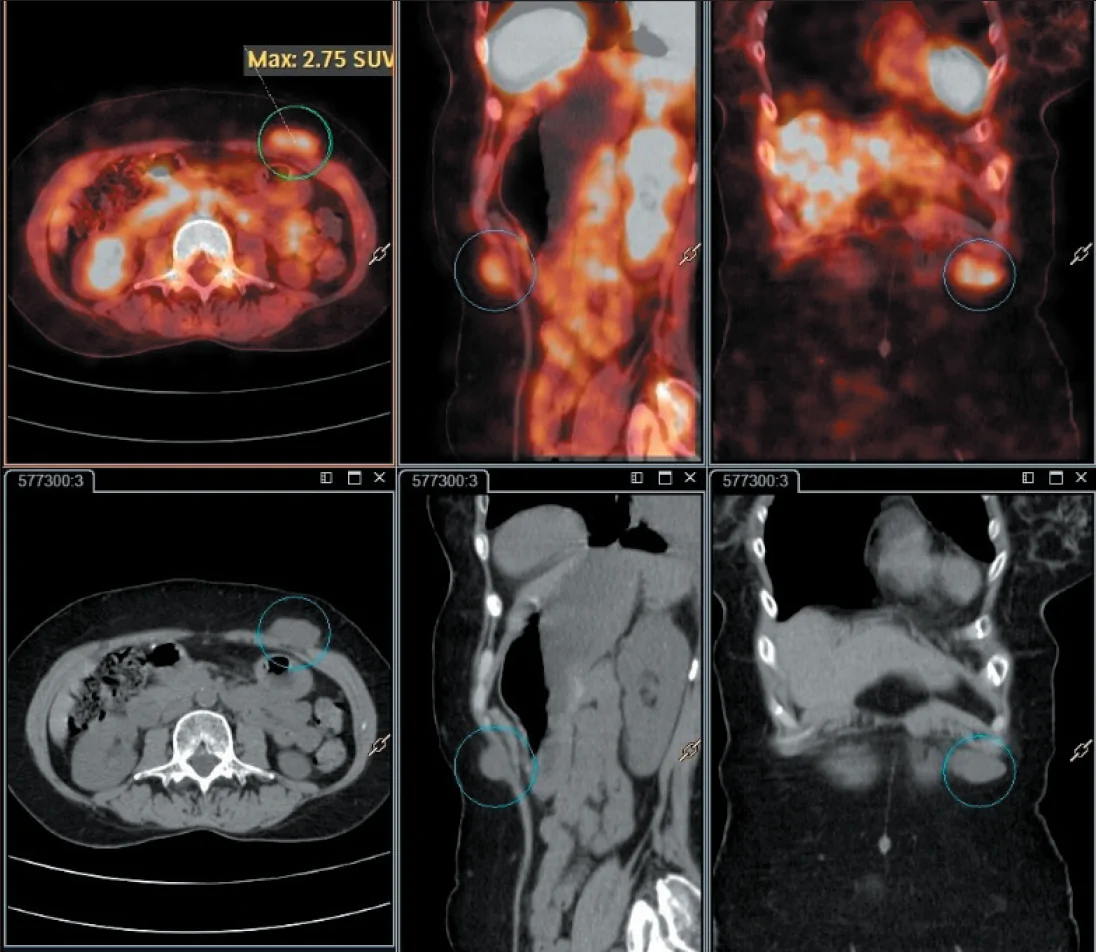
PET CT showing a metabolically active subcutaneous fat stranding was noted in the left hypochondrial region.
